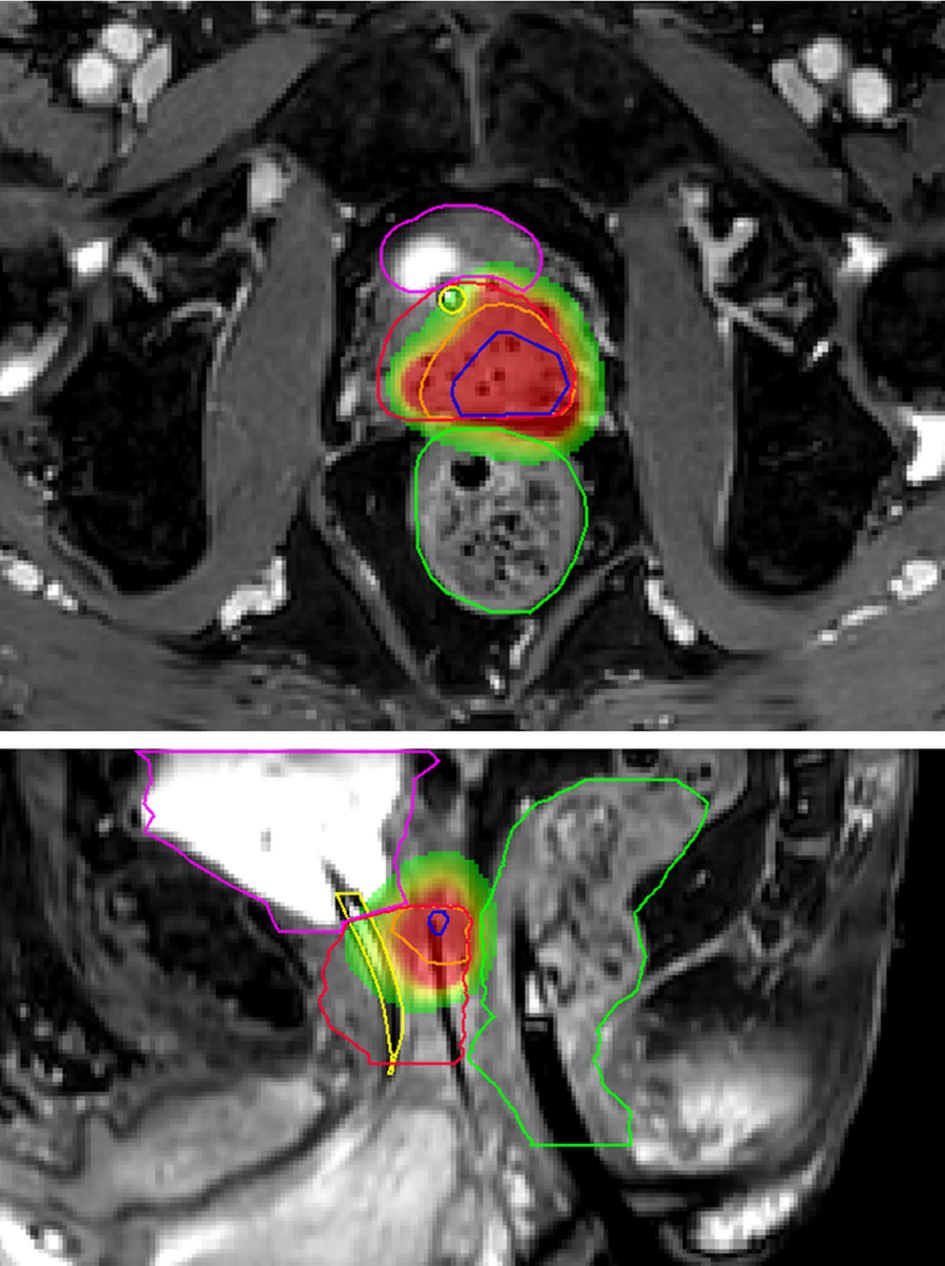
Dose distribution second MRI-guided focal salvage HDR-BT Transversal plane (upper MR image) and sagittal plane (lower MR image) showing delineations of the bladder (purple), urethra (yellow), rectum (green), prostate (red), gross tumor volume (GTV, blue), and clinical target volume (CTV, orange) in the left dorsal peripheral zone. The images show signal voids from the catheters and previously implanted iodine 125 seeds. On the sagittal image, one of the catheters is visible within the CTV. Radiation dose is displayed in colors, with red representing 19Gy and green representing 9.5Gy. HDR-BT: high-dose-rate brachytherapy; MRI: magnetic resonance imaging
